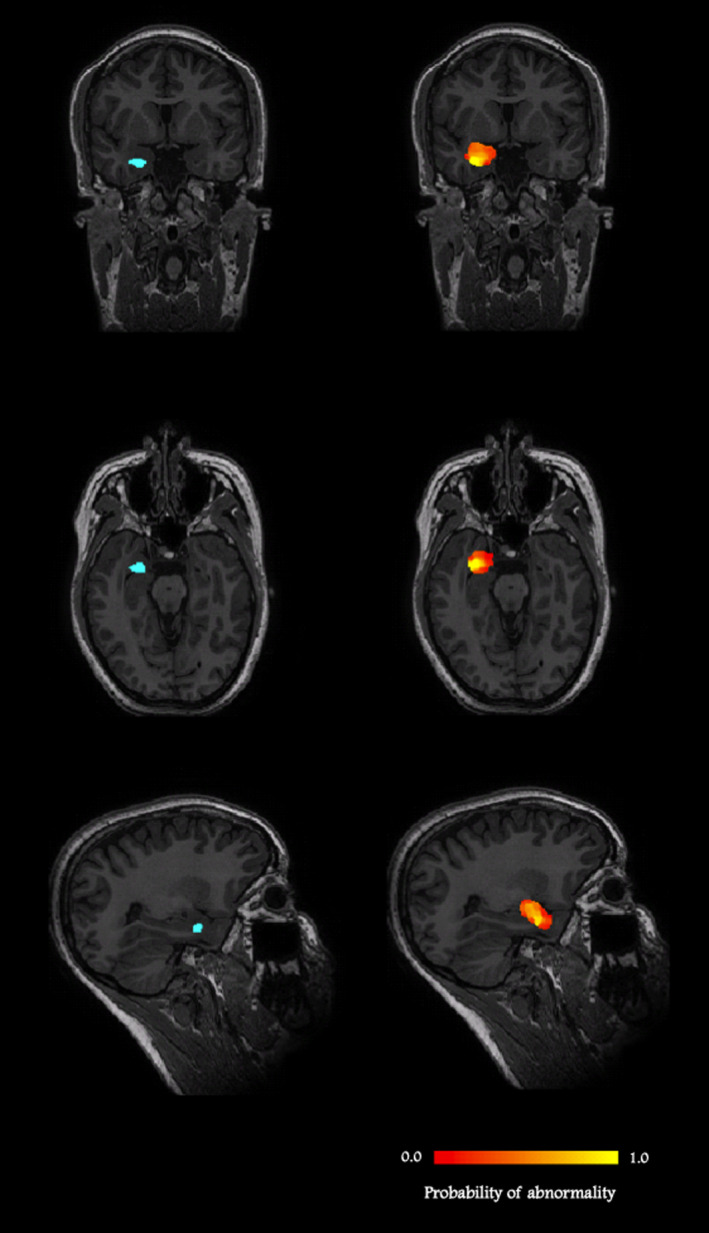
An MRI‐positive case: a 27‐year‐old female patient, with right amygdala dysembryoplastic neuroepithelial tumor. Images on the left show the manually drawn lesion mask in blue, whereas the images on the right show the probabilistic abnormality map detected by C2_vox_ in red (lowest probability) to yellow scale (highest probability). The lowest and highest probabilities of abnormality found in the lesional area detected in this case were 3% and 91%, respectively, with a mean over the lesion of 36%
